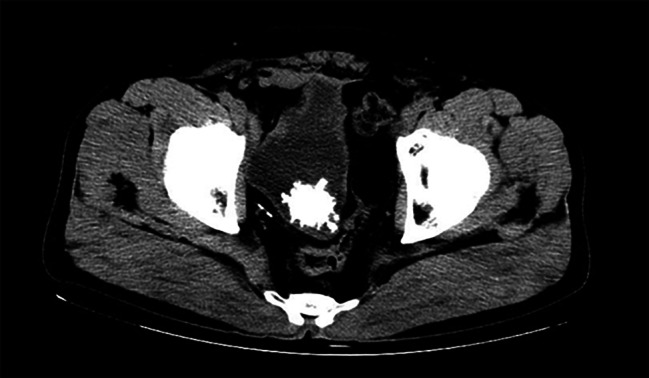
Cross view of computed tomography abdomen and pelvis shows large stone in the neobladder
